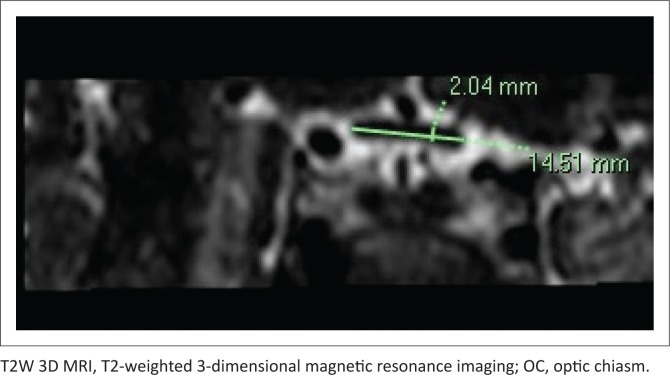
Reformatted coronal T2W 3D magnetic resonance imaging demonstrating the measurement technique of the optic chiasm width and height.
